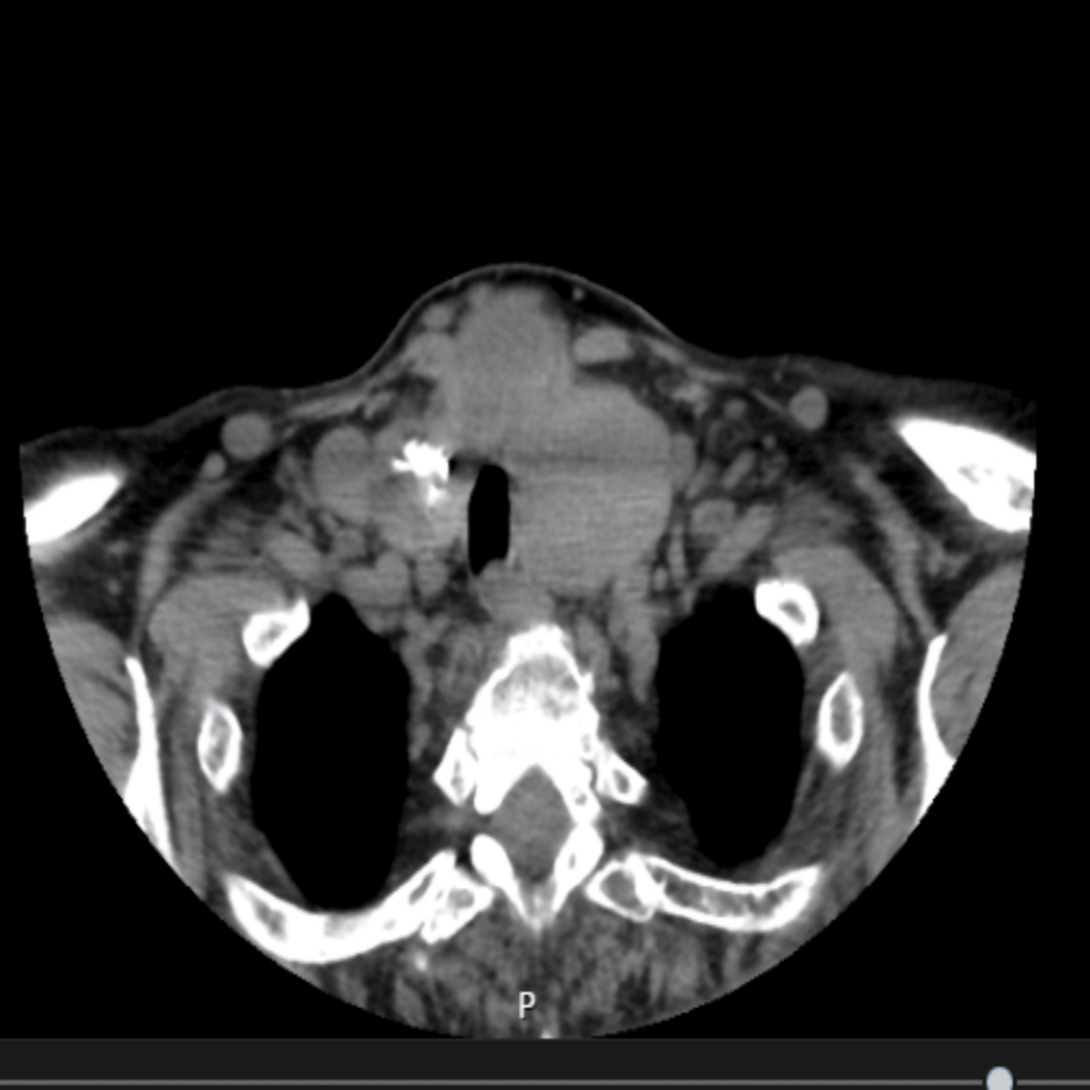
Thoracic computed tomography exhibiting a substernal goiter with tracheal deviation
